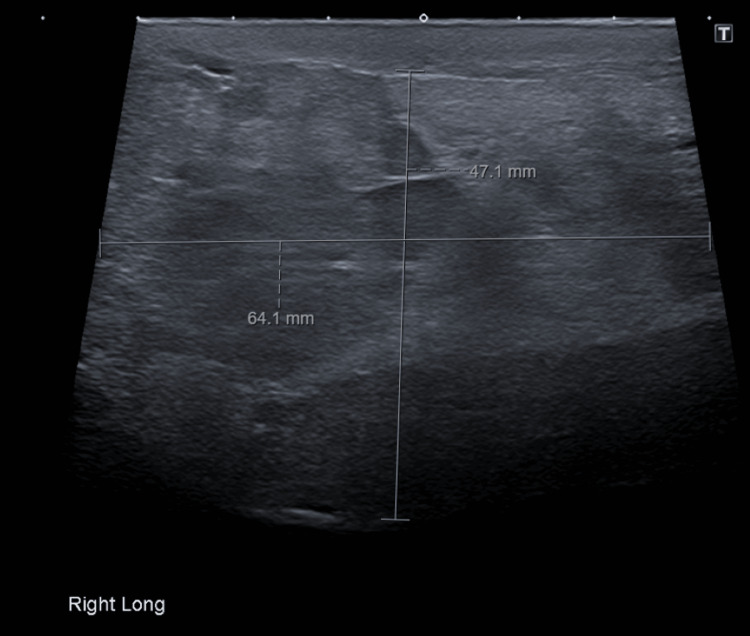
Case 2: The ultrasound showing a hyperechoic lesion in the right hemiscrotum.
